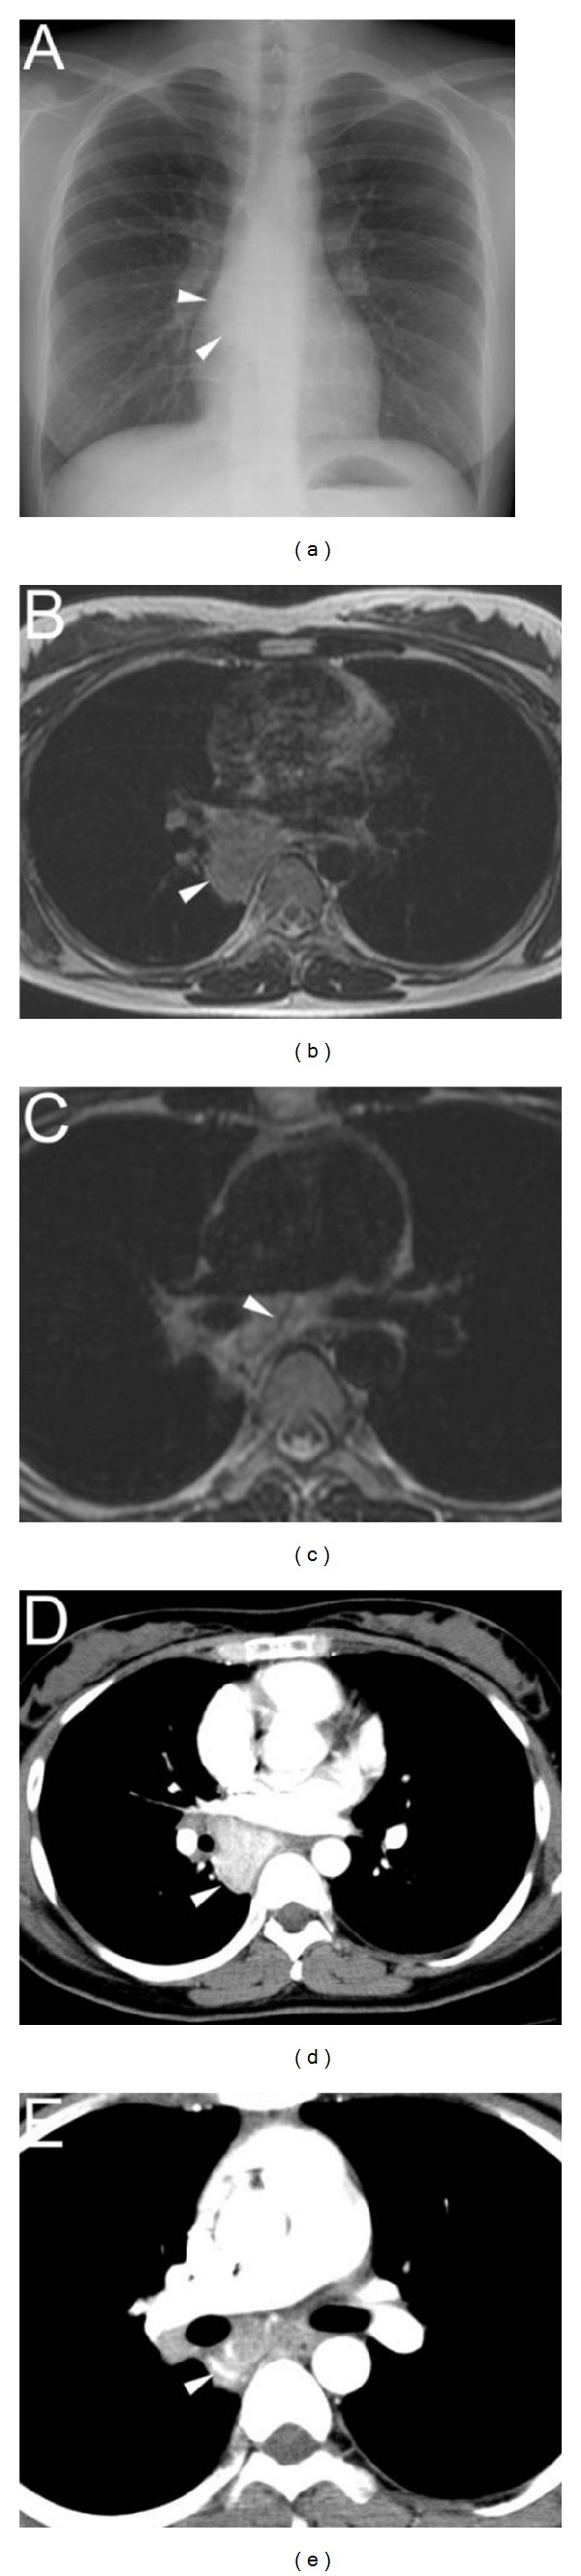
(a) Chest X-ray image showed a mass shadow in the cardiac silhouette obscuring the azygoesophageal line (arrowhead). (b) and (c) Magnetic resonance imaging (MRI) revealed a mass in the subcarinal azygoesophageal recess. The mass was hyperintense on T2-weighted images (arrowhead) (b). Flow voids (arrowhead) were detected within the mass (c). (d) and (e): On contrast-enhanced computed tomography (CT) scan, the mass was enhanced homogeneously and intensely (arrowhead) (d), and enlarged vessels were detected just cephalad to the tumor (arrowhead) (e).
